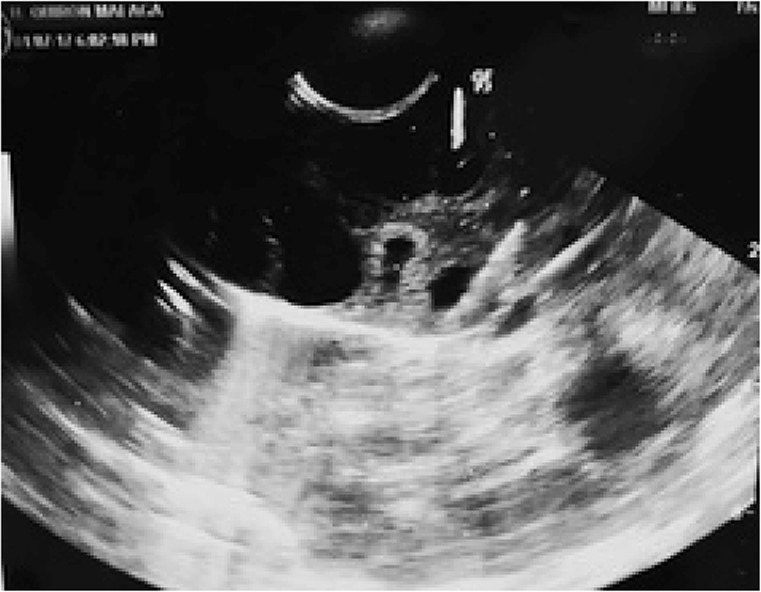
Ultrasound image during guided needle follicular aspiration
